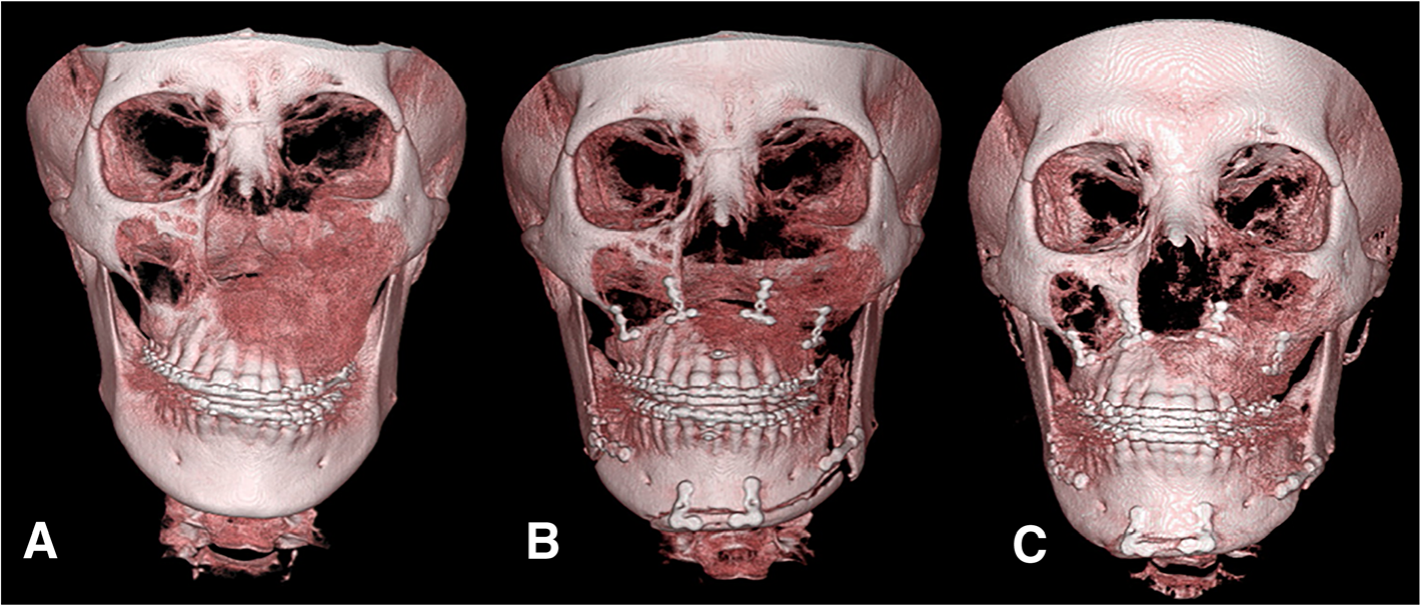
3D CT image of patient no. 1 preoperative (a) immediately postoperative (b) and 1 year postoperative status (c) showing improvement in occlusal canting following Le Fort I and wing osteotomy on the mandibular body and chin with rigid fixation
